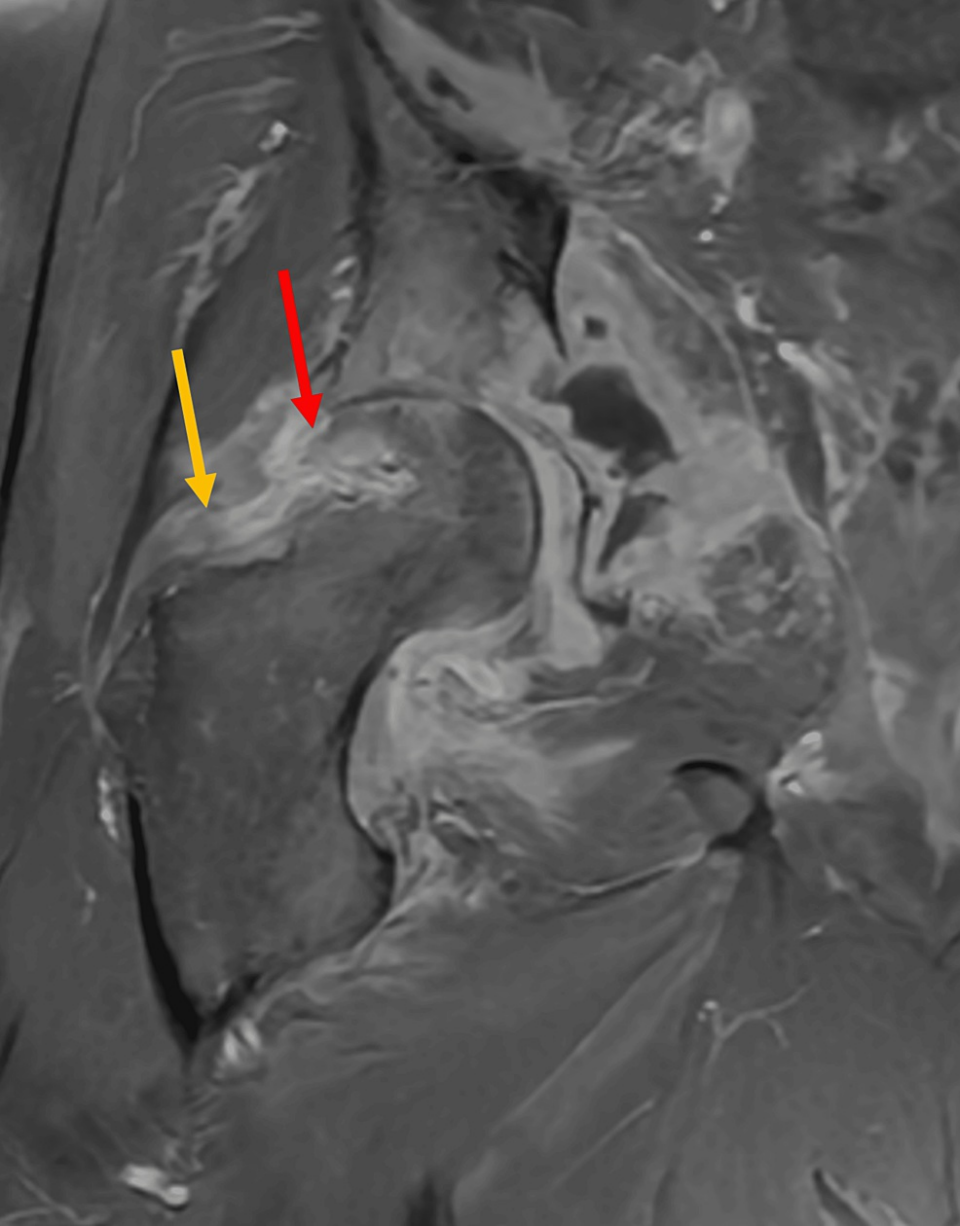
MRI of the right hip. Stable appearance of septic arthritis (yellow arrow). Interval regression of osteomyelitis to the femoral head and neck (red arrows). The grossly stable appearance of collections distribution with interval reduction in the number of adductor collections.
